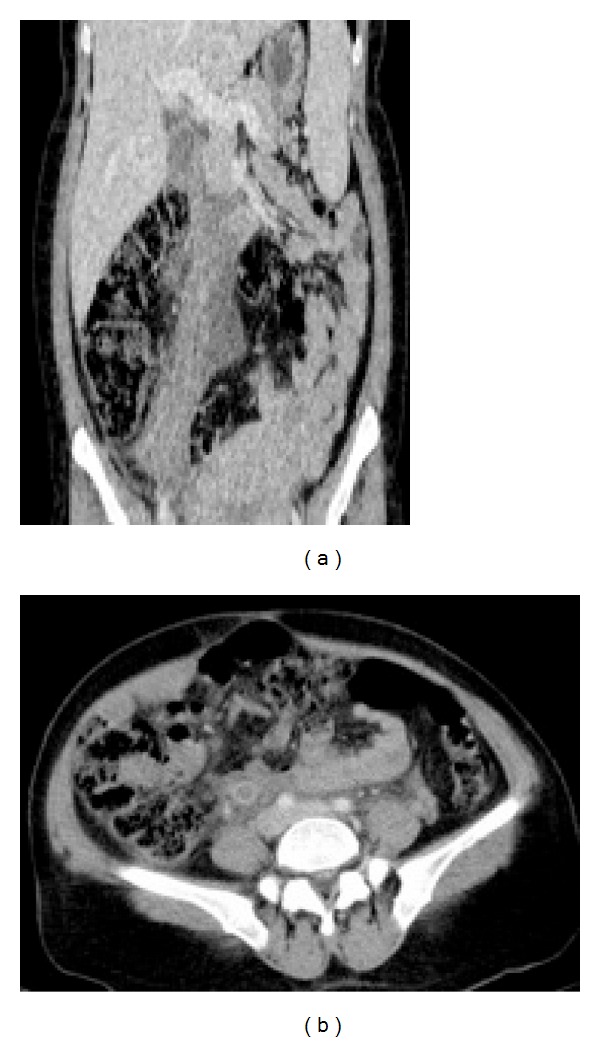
In coronal (a) and axial (b) CT images; enlarged ovarian vein, inflamed perivascular area, and thrombus in ovarian vein (OV) and inferior vena cava (IVC).
